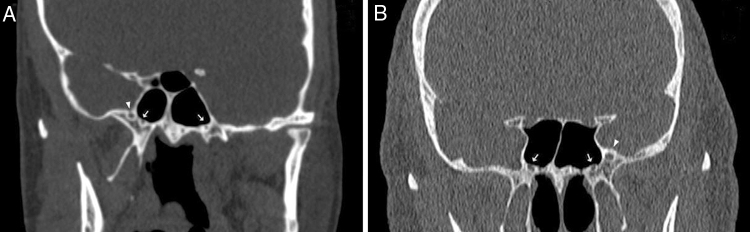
(A) Coronal CT image of 19-year-old woman showing unilateral right foramen rotundum (arrowhead, she had no left foramen rotundum) and the vidian canals (thick arrow); (B) coronal CT image of 68-year-old man showing unilateral left foramen rotundum (arrowhead, he had no right foramen rotundum) and the vidian canals (thick arrow).
